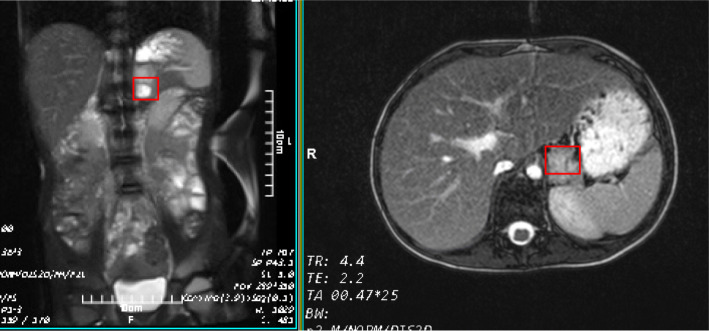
Abdominal magnetic resonance imaging (MRI) which depicts a left adrenal gland nodule (red boxes, over 3 cm diameter)
